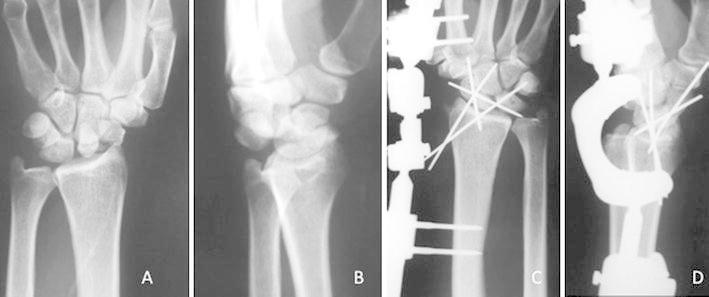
Posteroanterior (a) and lateral (b) radiographic views of the wrist of a 45-years-old woman showing a trans-scaphoid perilunate fracture dislocation. Postoperative posteroanterior (c) and lateral (d) radiographs of the wrist after open reduction, external fixation and application of K-wire, demonstrating restoration of normal carpal alignment
